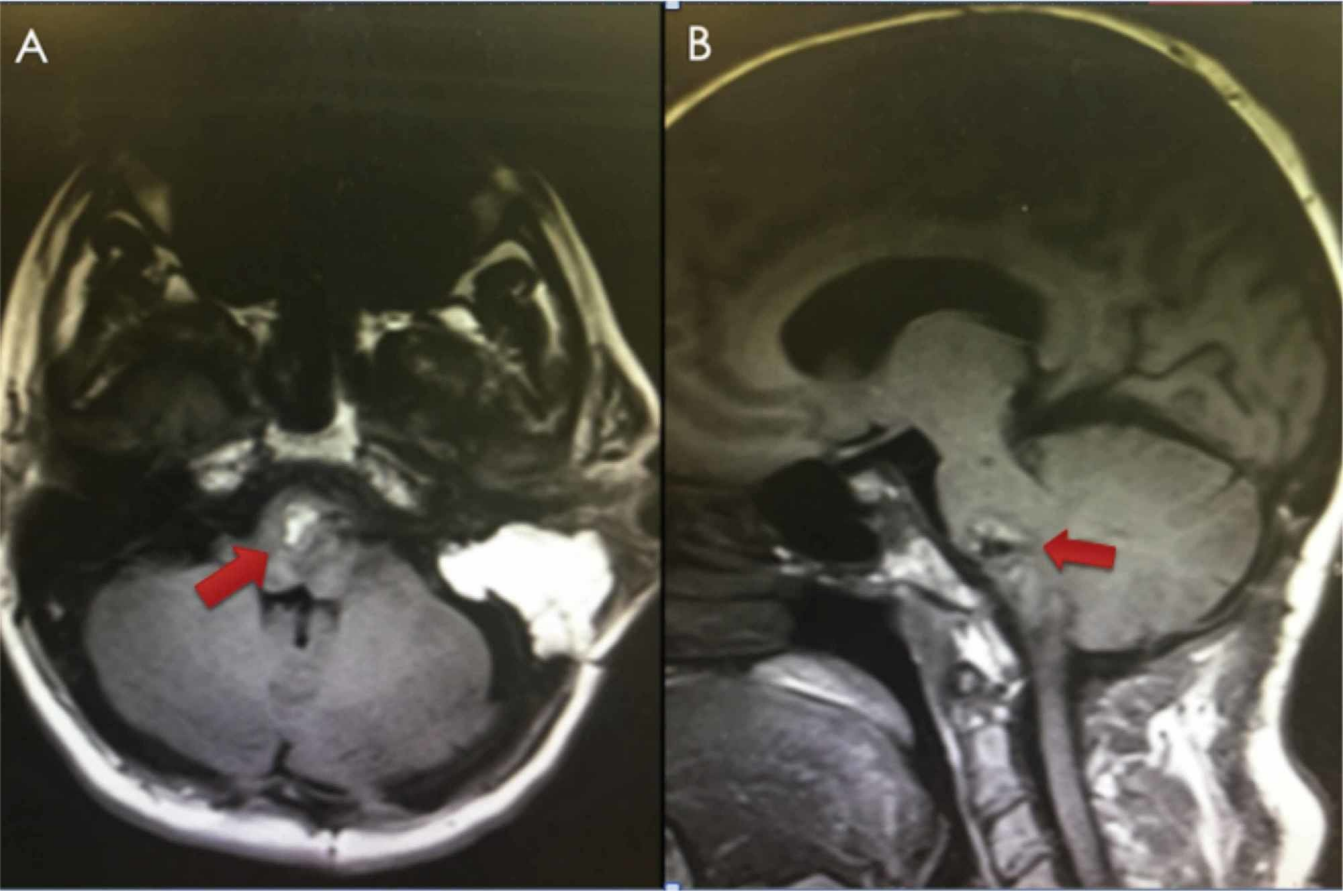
Postoperative MRI (T1W without contrast) showing satisfactory resection of the pontomedullary lesion with a fat graft in the resection cavity (red arrows). MRI: magnetic resonance imaging
